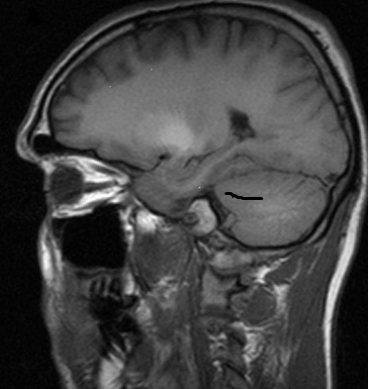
Brain MRI, T-1 sagittal view showing high signal in globus pallidus.
